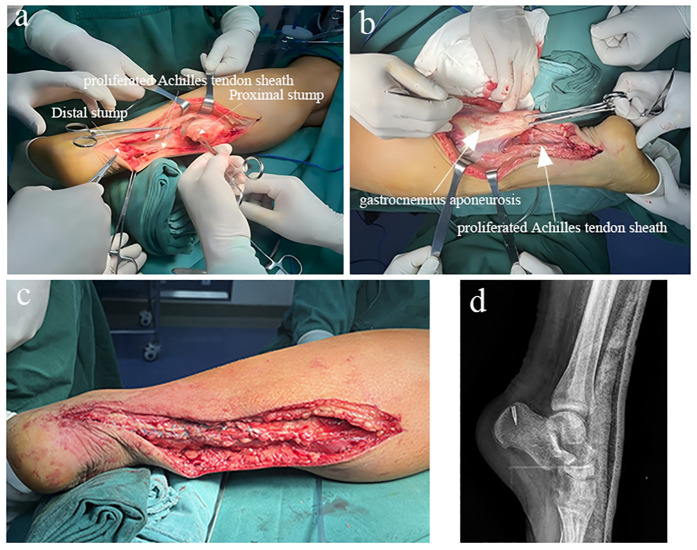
(a,b) Intraoperative exploration showing intact gastrocnemius aponeurosis and achilles tendon sheath with marked scar hyperplasia, and shortening of the Achilles tendon stumps. (c) The gastrocnemius aponeurosis flap and proliferated Achilles tendon sheath were folded and sutured into a cord-like structure resembling the native Achilles tendon. (d) Postoperative digital radiograph demonstrating appropriate placement of the suture anchor.
